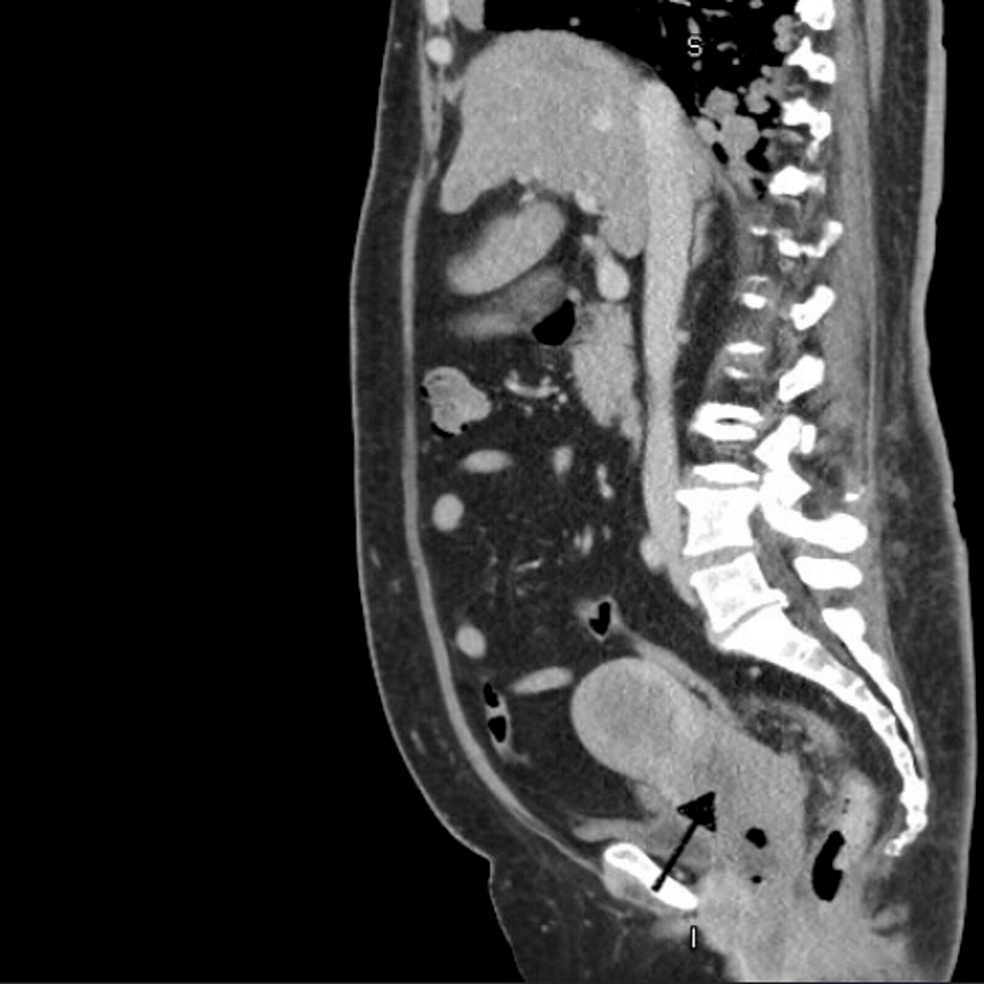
CT abdomen pelvis (sagittal view) showing soft tissue mass involving the cervix with nonhomogeneous density suggesting an underlying cervical mass (black arrow)
